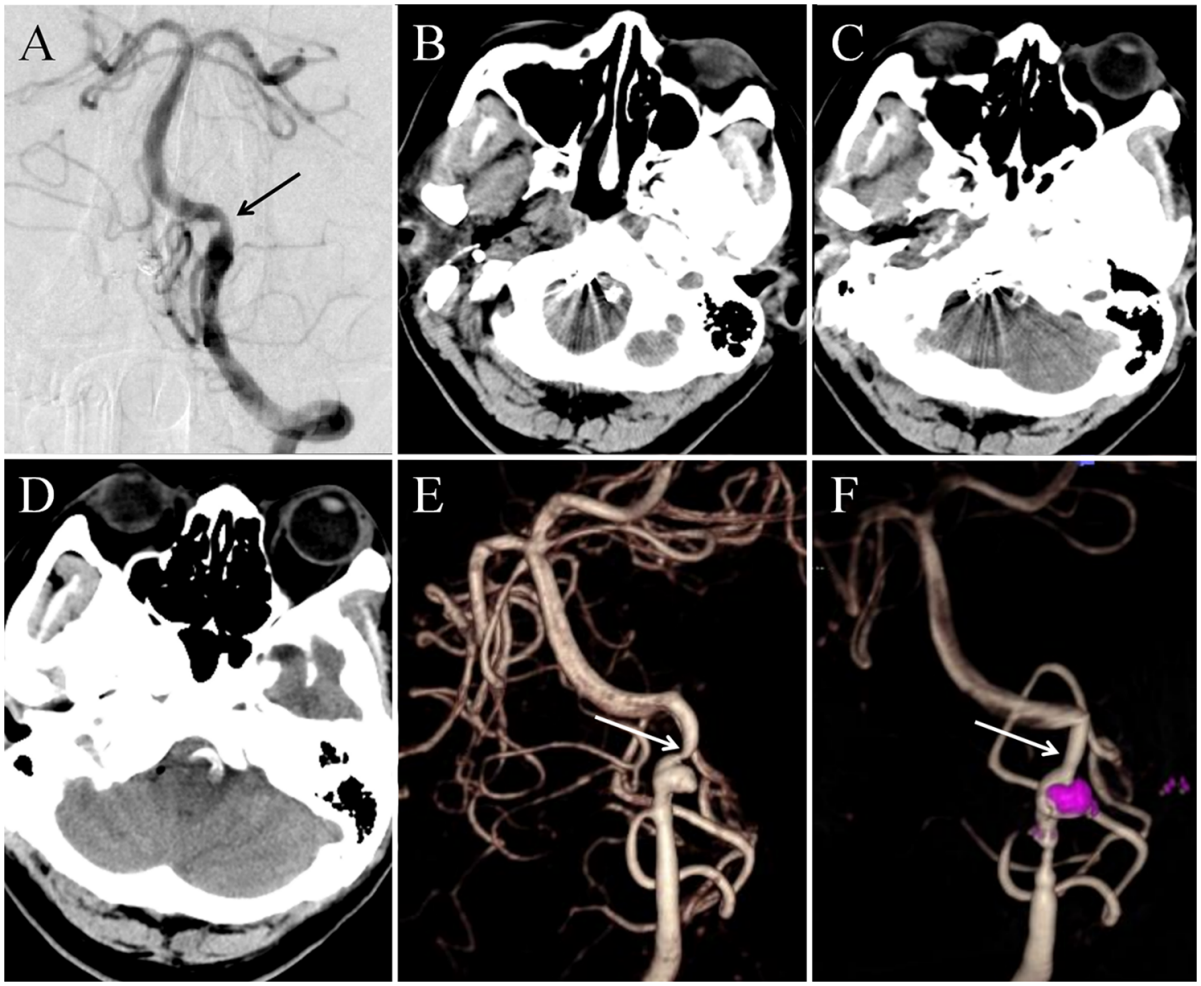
(A) Postoperative angiography indicated that the distal stenosis of the contralateral vertebral artery had been relieved. (B–D) Postoperative head CT examination revealed that the stent crossed the V4 segment of the bilateral vertebral artery, and no new hemorrhage and cerebral infarction were found. (E,F) Preoperative and postoperative 3D reconstruction showed that vascular stenosis was significantly improved, the single stent completely covered the dissection and stenosis of bilateral vertebral arteries, and basilar aneurysm blood flow was unobstructed.
